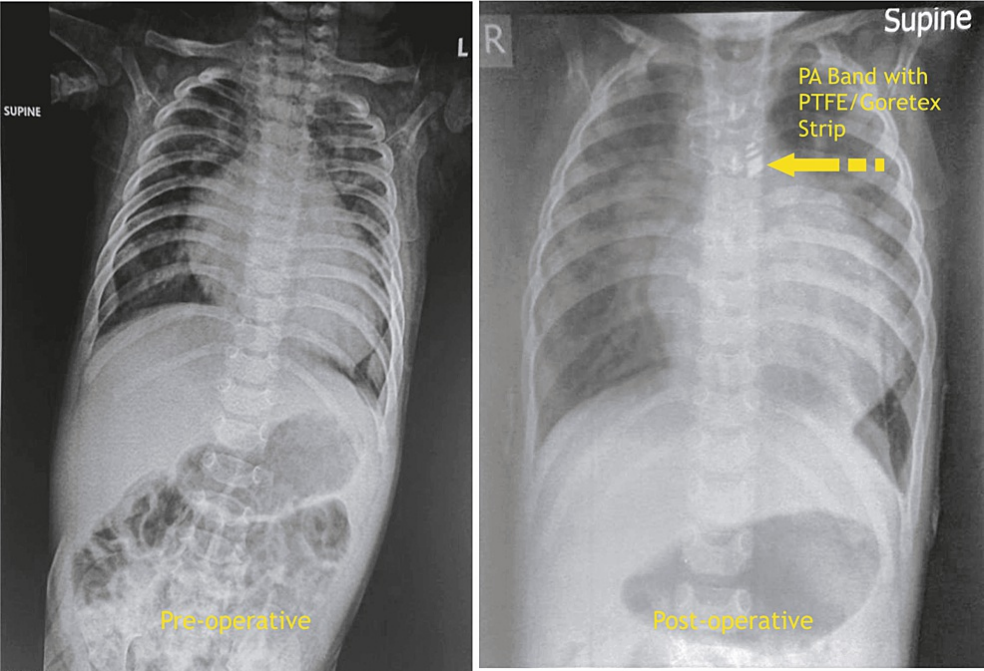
Preoperative and postoperative chest X-ray of a 16-month-old patient with pulmonary artery band in situ PA - pulmonary artery, PTFE - polytetrafluoroethylene
